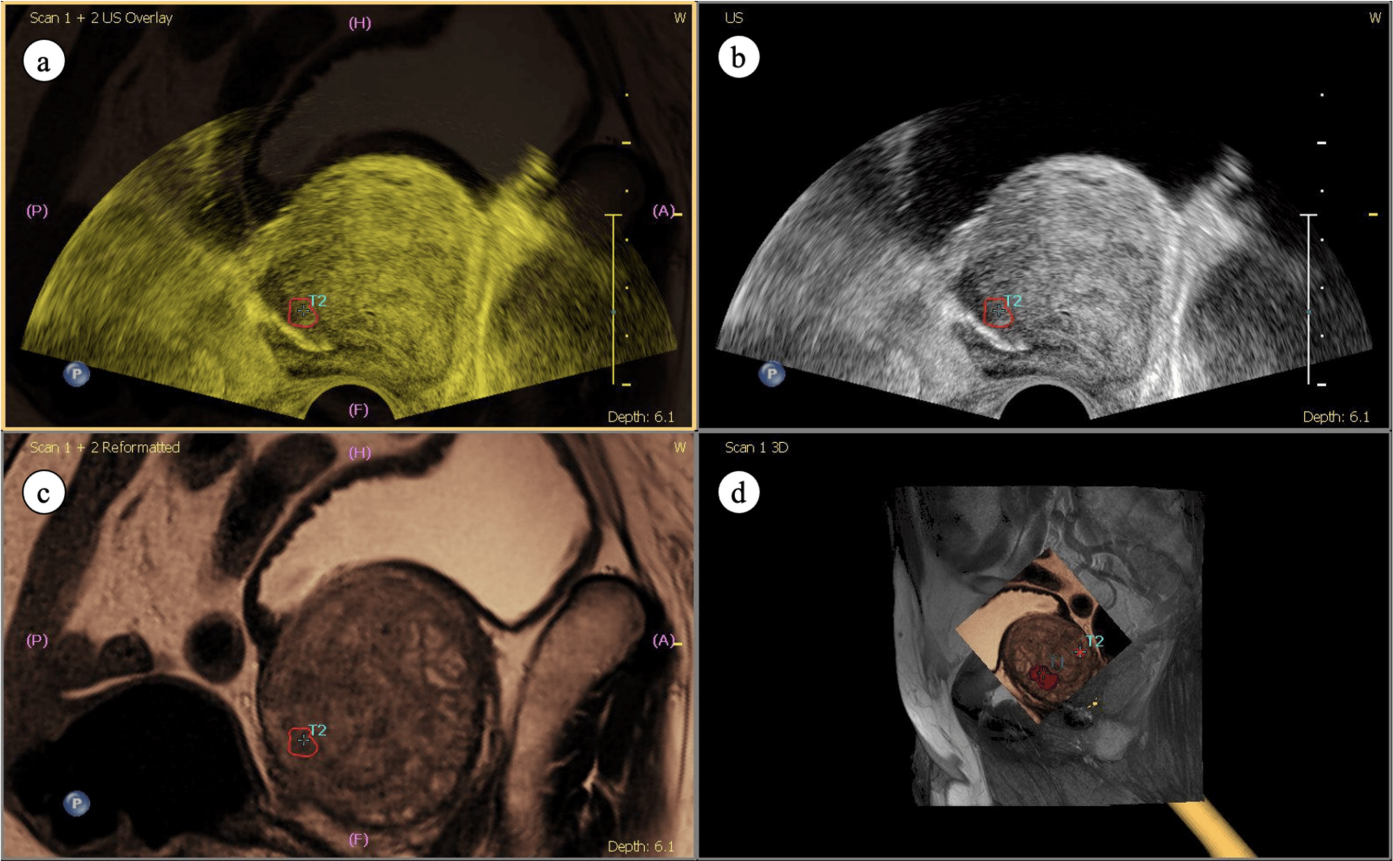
MRI/TRUS fusion images (a) blend overlay of both (b) ultrasound and (c) MR images, showing accurate fusion. The targeted lesion is indicated by the red marking. (d) Reconstructed three dimensional image according to real-time probe orientation. Abbreviations: MRI=magnetic resonance imaging; TRUS=transrectal ultrasound
